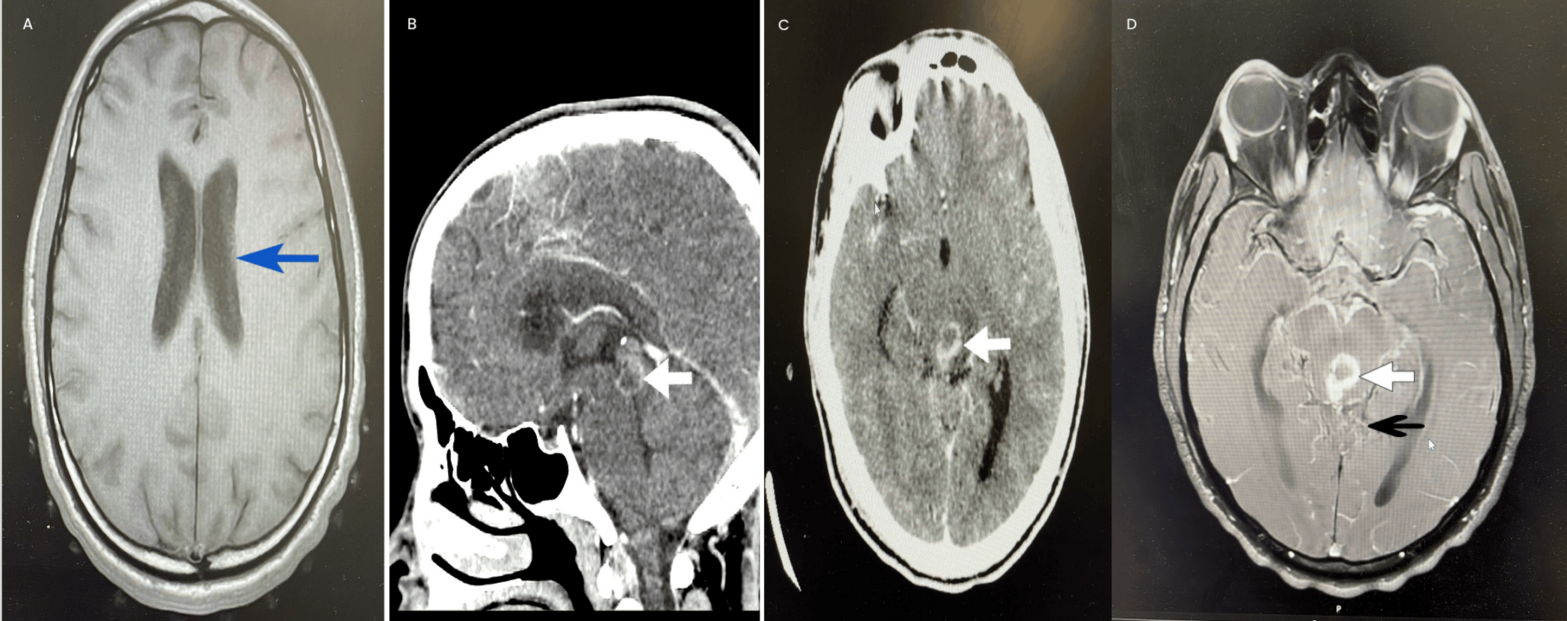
(A) Axial CT head showing dilatation of the lateral ventricles, consistent with early hydrocephalus. (B) Sagittal contrast-enhanced CT demonstrating a 17 × 16 mm peripherally enhancing lesion centered on the cerebral aqueduct, with a central non-enhancing component. (C) Axial CT at the level of the midbrain showing the same lesion in cross-section. (D) Post-contrast T1-weighted axial MRI demonstrating intense enhancement of the circular periaqueductal lesion, with subtle leptomeningeal enhancement within the quadrigeminal and prepontine cisterns. CT: computed tomography, MRI: magnetic resonance imaging, blue arrow: dilated lateral ventricle, white arrow: solitary peripherally enhancing mass lesion, black arrow: leptomeningeal enhancement
